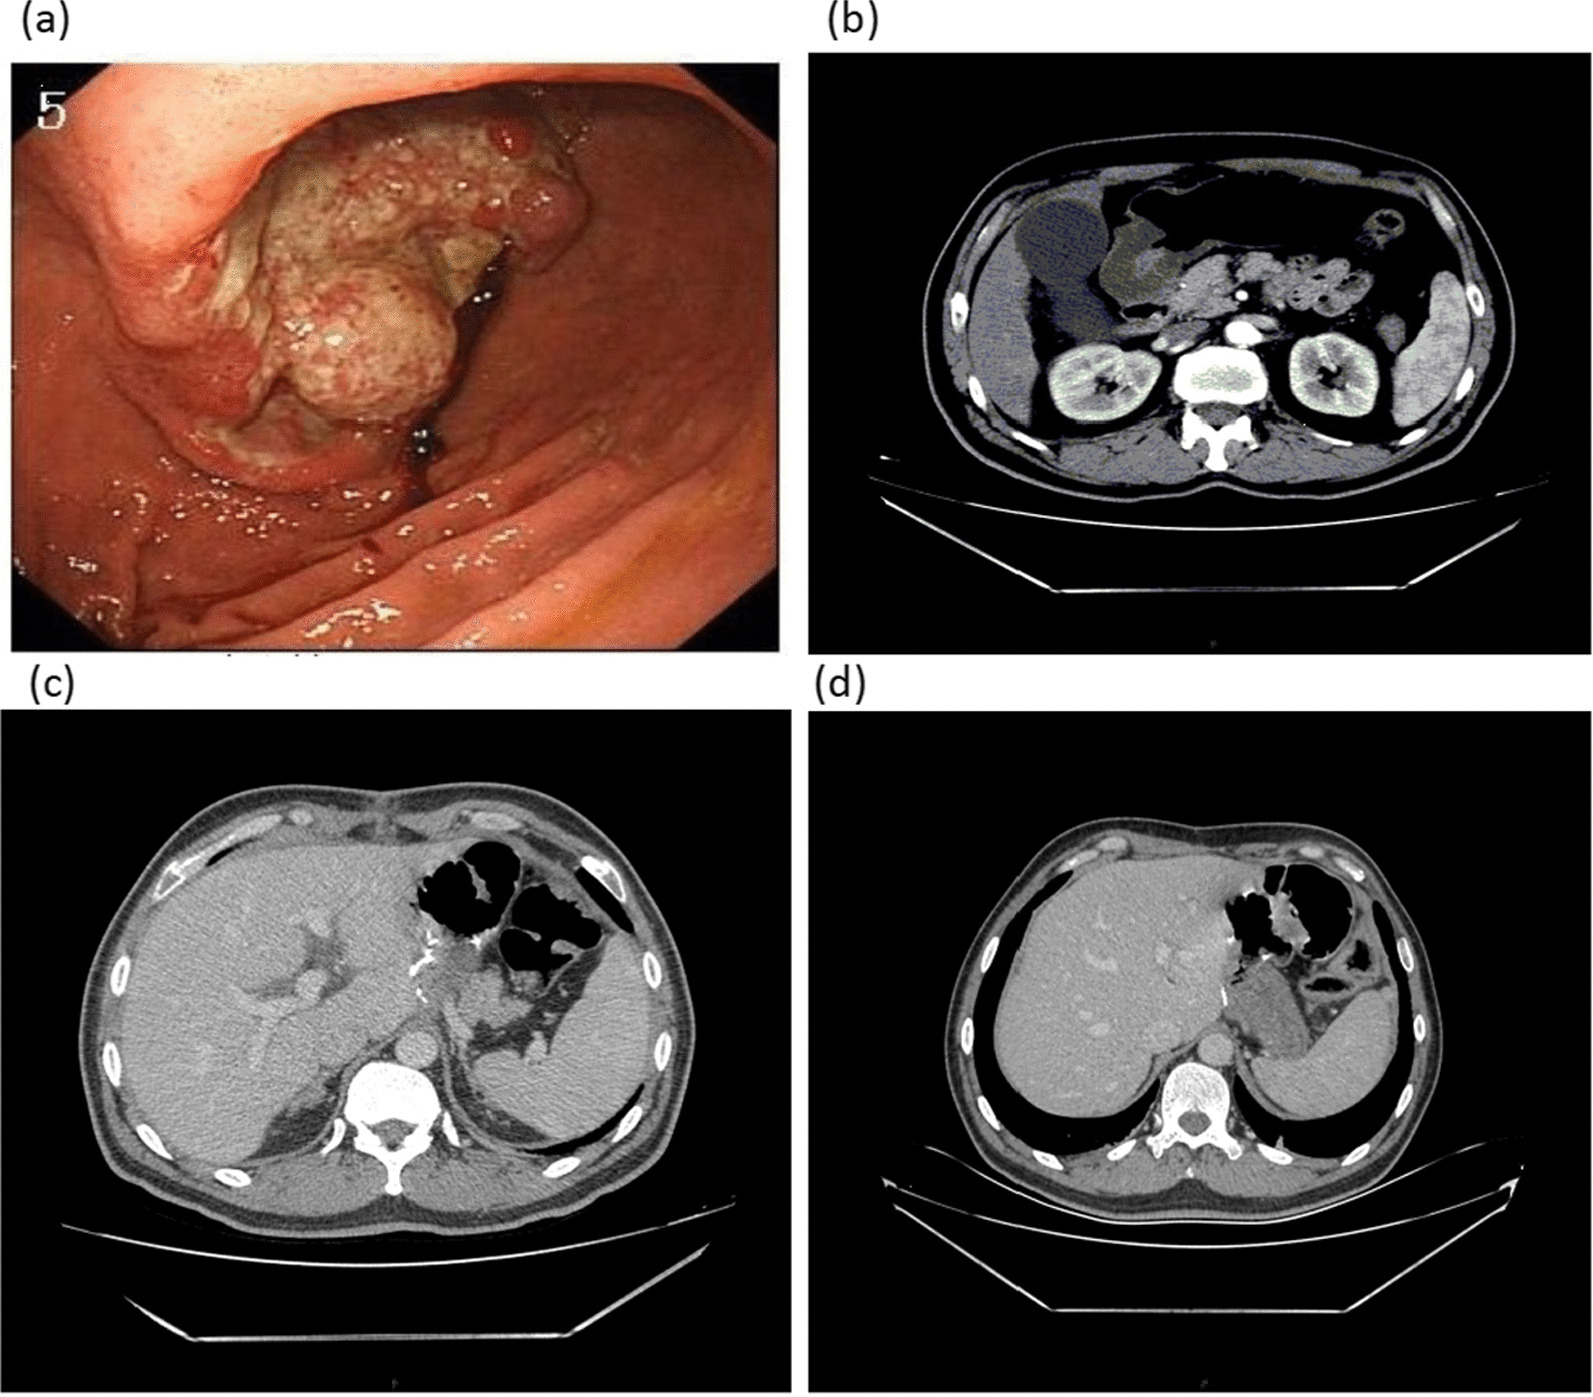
Representative cases of long-term survivors. a The patient’s tumor was located in the gastric body before treatment. b Patient after neoadjuvant therapy. c Postoperative condition of patients. d Patient’s recent CT results
